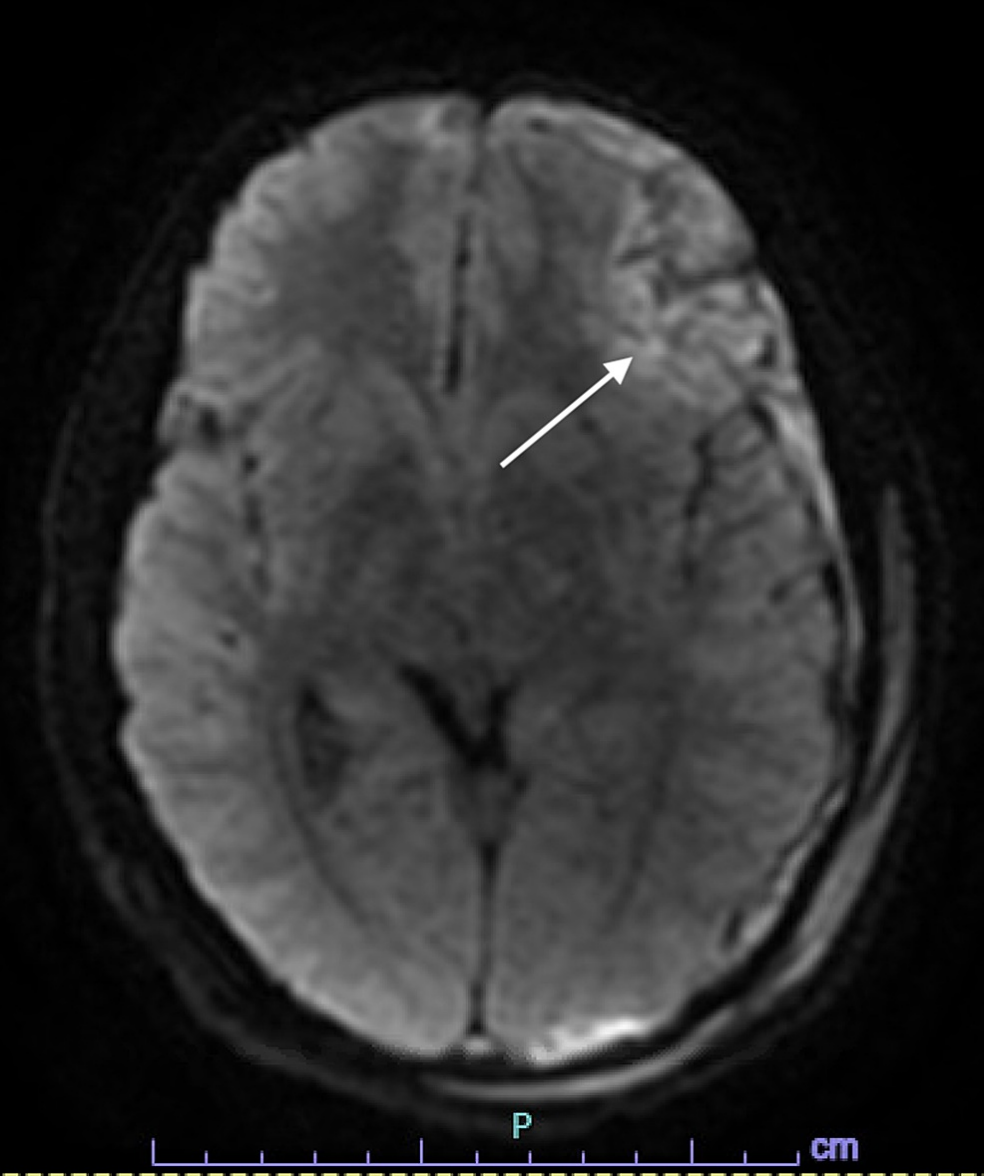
MRI brain diffusion-weighted image. A 4.0 cm × 2.9 cm left frontal intraparenchymal hemorrhage (indicated by the arrow) with subarachnoid hemorrhage and subdural hematoma components was evident.
